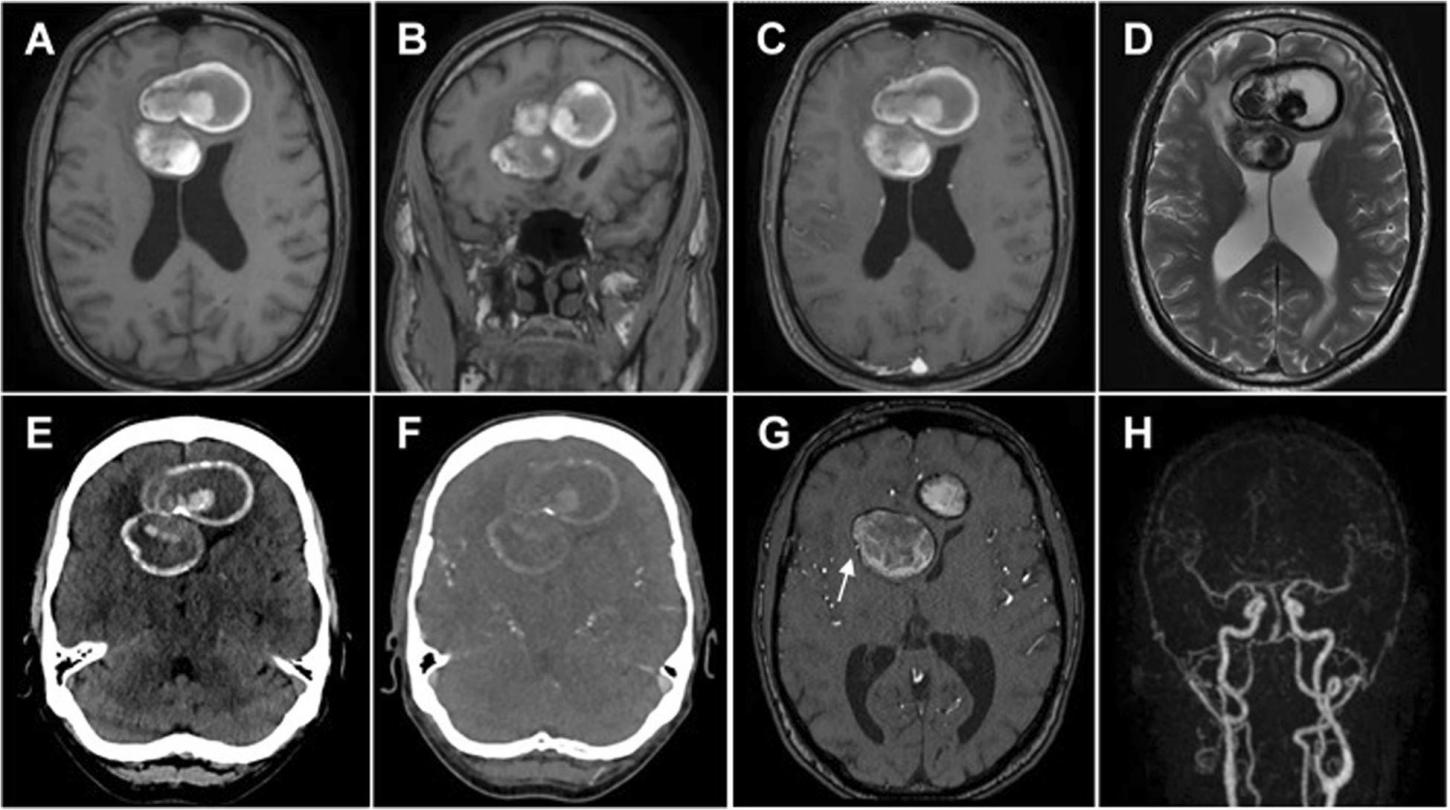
Preoperative imaging. Preoperative imaging showing in the cranial MRI a bilobed and bihemispheric huge mass (54 and 40 mm in diameter) of both frontal lobes with a crescent shaped hyperintensity of the lesion wall in the T1-weighted frame (a, b), without rim enhancement of the lesion wall or intraluminal flow void after administration of Gadolinium (c) and with perifocal edema in the T2-weighted frame (d). Non-enhanced cranial CT demonstrated a partially hyperdense mass lesion with partial peripheral and intralesional calcifications as well as intramural hemorrhage and slight perifocal edema (e), but without contrast enhancing ring after contrast administration (f). TOF-MRI (g) showed the right ACA at the lateral wall of the lesion (white arrow). TWIST-MRI (h) demonstrated neither contrast enhancement nor intraluminal filling of both masses. MRI: Magnetic resonance imaging, CT: Computed tomography, TOF: Time-of-flight, ACA: Anterior cerebral artery, TWIST-MRI: Time-resolved angiography with interleaved stochastic trajectories MRI
